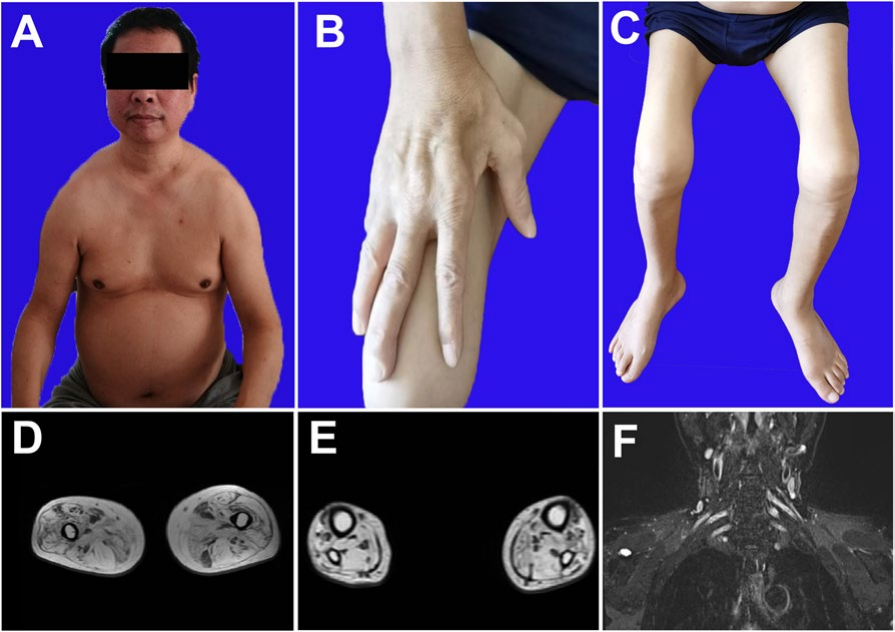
Clinical and MRI findings of patient 1. The patient exhibited scapular muscle atrophy, resulting in sloping shoulders (A), as well as significant atrophy of hand intrinsic muscles (B) and calf muscles (C). MRI revealed atrophy of the thigh (D) and calf (E) muscles with concurrent fat infiltration. The brachial plexus was hypertrophy and hyperintense (F)
